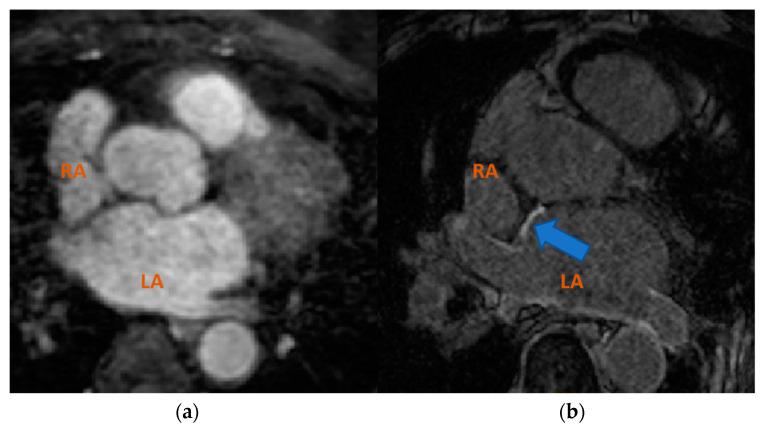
Axial view of different MRI sequences. (a) bSSFP and (b) LGE-MRI. The blue arrow points to the enhanced brightness of infarcted regions obtained in LGE-MRI. Atrial cavities are labelled as LA (left atrium) and RA (right atrium).
